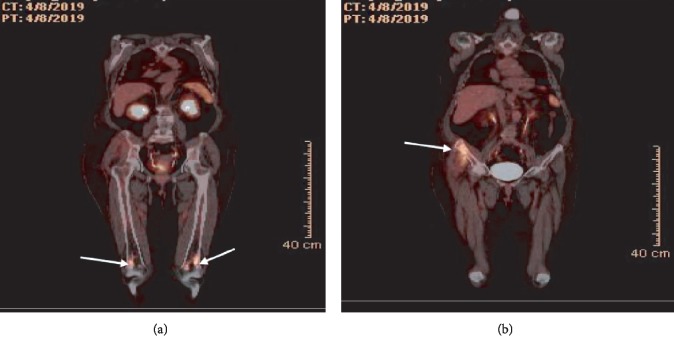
18-Fluorodeoxyglucose positron emission tomography (coronal views): ^18^FDG-PET scan showing FDG-avid sclerotic bone lesions in the distal femoral metadiaphysis bilaterally (a) as well as the right iliac bone involvement (b).
